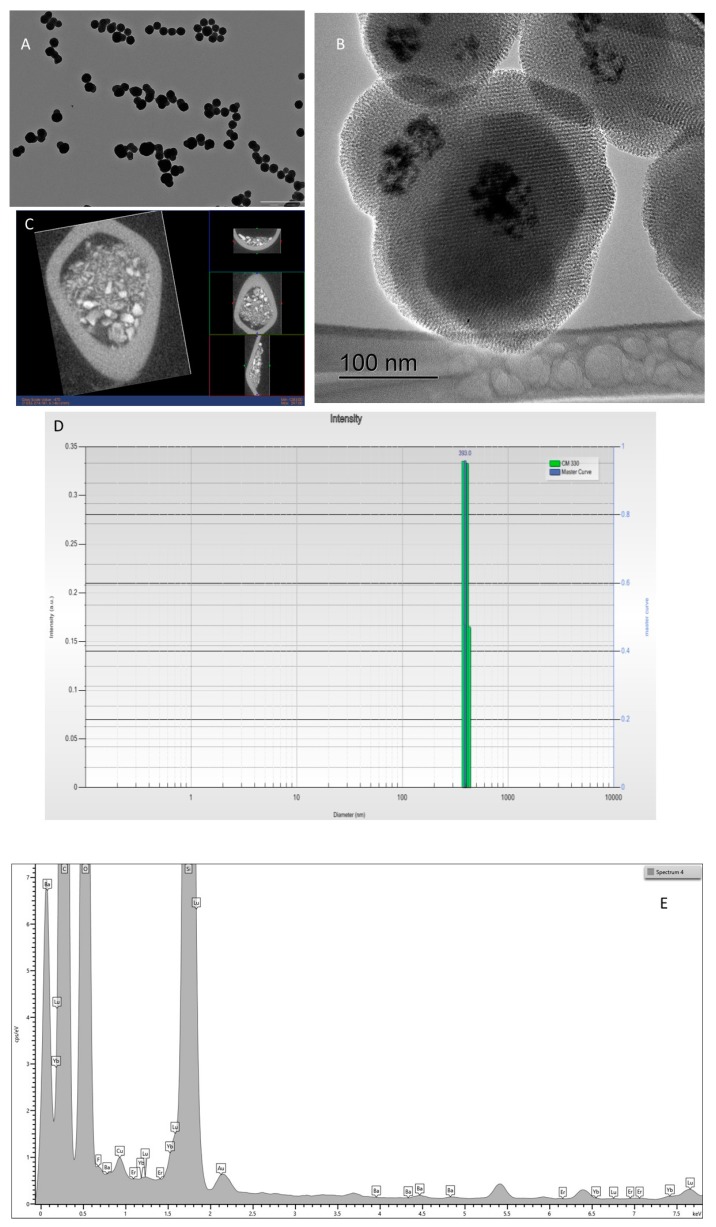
(A) TEM image of BaLuF_5_: Yb^3+^-Er^3+^@Ethane scale bar 1 µm (B) HRTEM of BaLuF_5_: Yb^3+^-Er^3+^@Ethane. (C) X-ray computed tomography of BaLuF_5_: Yb^3+^-Er^3+^@Ethane. (D) DLS of BaLuF_5_: Yb^3+^-Er^3+^@Ethane in EtOH. (E) EDX analysis of BaLuF_5_: Yb^3+^-Er^3+^@Ethane. Si, C, O, F, Ba, and Lu elements were detected.
